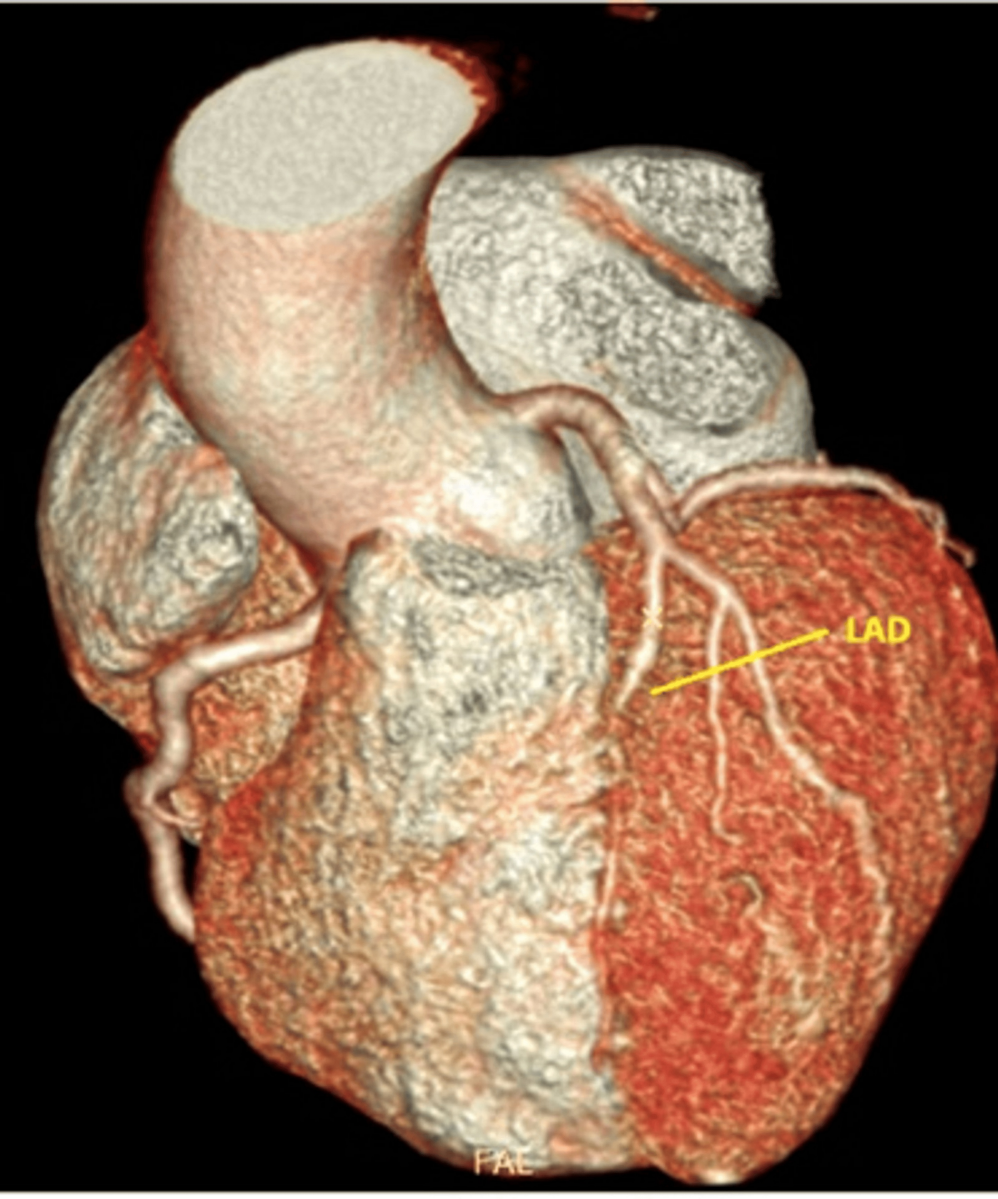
3D cardiac CT revealing a long-segment myocardial bridge in the LAD artery LAD, left anterior descending
